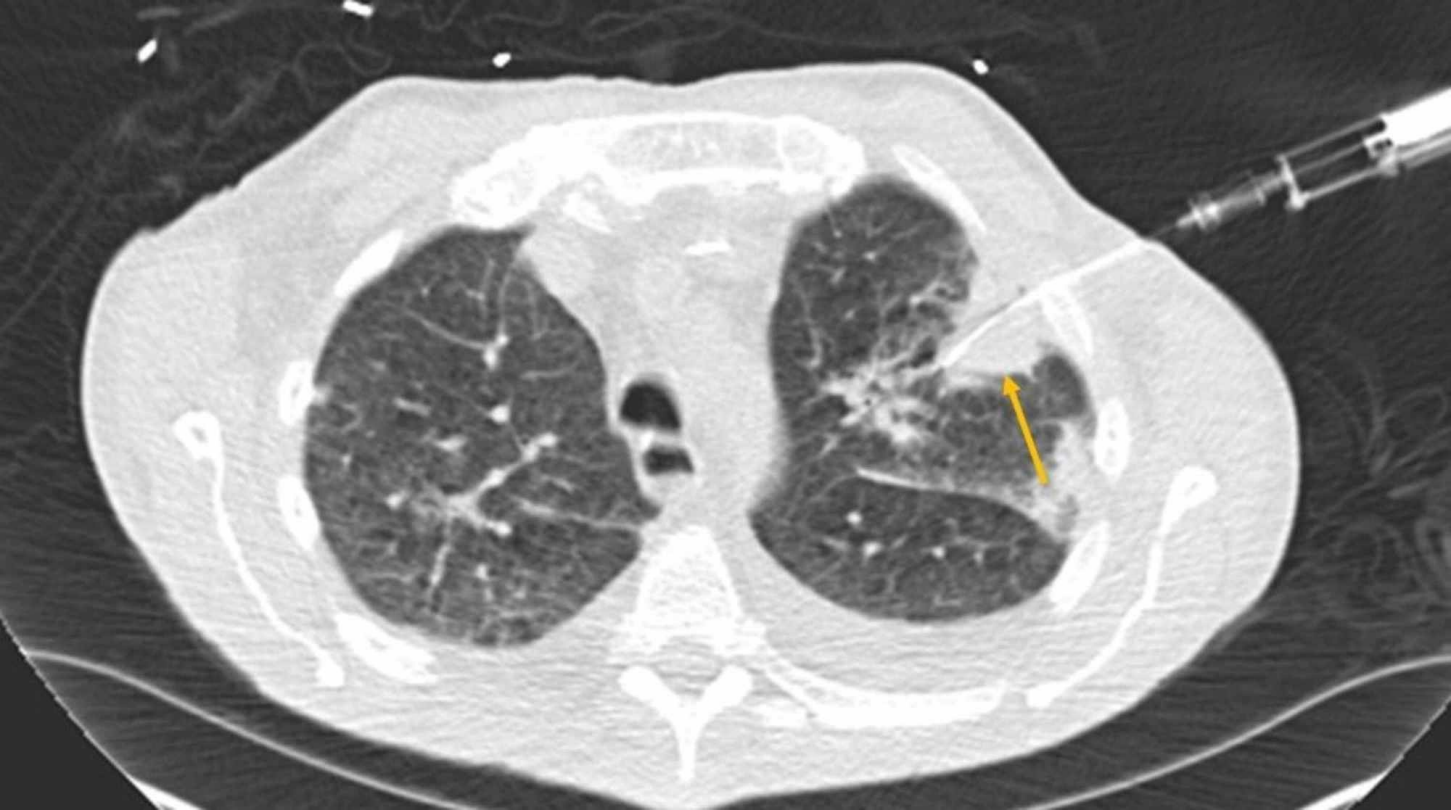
Axial chest CT CT-guided biopsy of a left upper lobe cavitary lesion (the yellow arrow) CT: computed tomography
